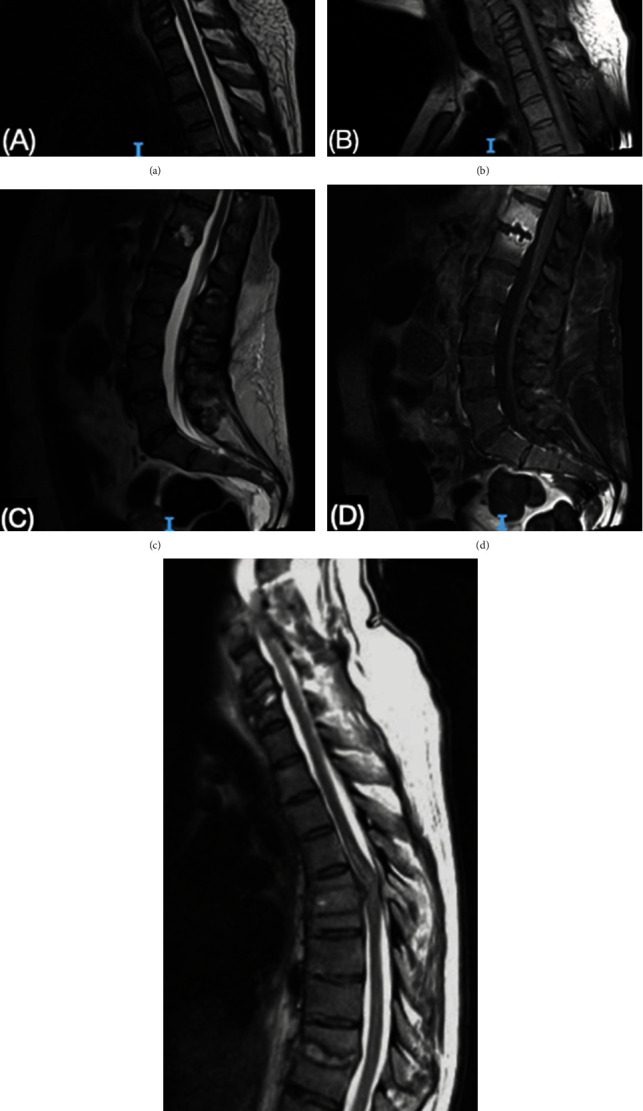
MRI showing the cervical, thoracic, and lumbar spine. (a, b) At the cervical spine, loss of the lordotic angle is demonstrated; destruction of the 6th cervical vertebrae is noted. In C3-C4, there is a collapse of the disc with changes in the signal intensity of the vertebral endplates, which are hyperintense in STIR T2 and isointense in T1 as well as an epidural abscess of 27 × 4.5 mm and encompasses the entire topography of C3 and C4, displacing and compressing the spinal cord. (c, d) At the level of the T12 and L1 vertebral bodies, respectively, in their lower and upper endplates, there is an erosive lesion of 17 × 18 mm involving the intervertebral disc, hyperintense on T2, and hypointense on T1, presenting wall enhancement following contrast. (e) The morphology and height of vertebral bodies T6 and T7 presented morphology alterations due to the destruction of the lower and upper vertebral endplates, respectively, associated with an expansive mass including the intervertebral disc, noted as hypointense on T1 and enhanced on T1 FatSat with gadolinium, and these changes are also observed in the epidural space, surrounding the spinal cord.
